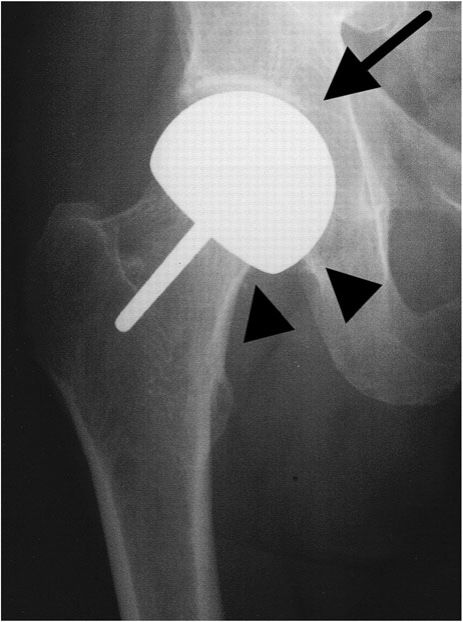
AP radiograph. Cementless resurfacing hemiarthroplasty. Only the femoral head is replaced (*arrowheads*), which articulates with the native acetabulum (*arrow*)
